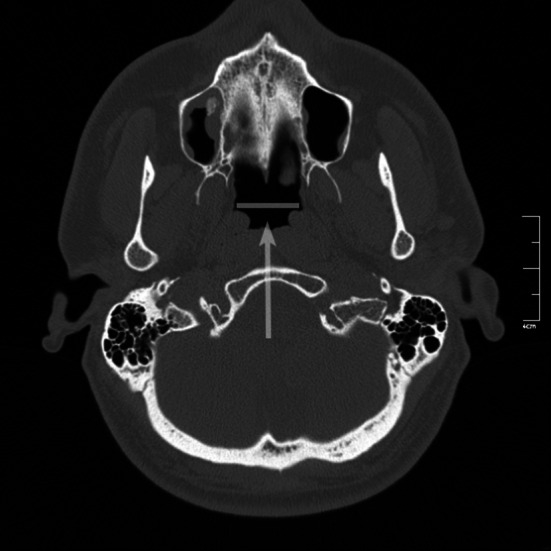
Craniofacial computed tomography scan. Pharyngeal openings of the auditory tube were determined on the axial plane. The *cursor* (*arrow*) was placed in the middle of a line joining the openings of the auditory tube
